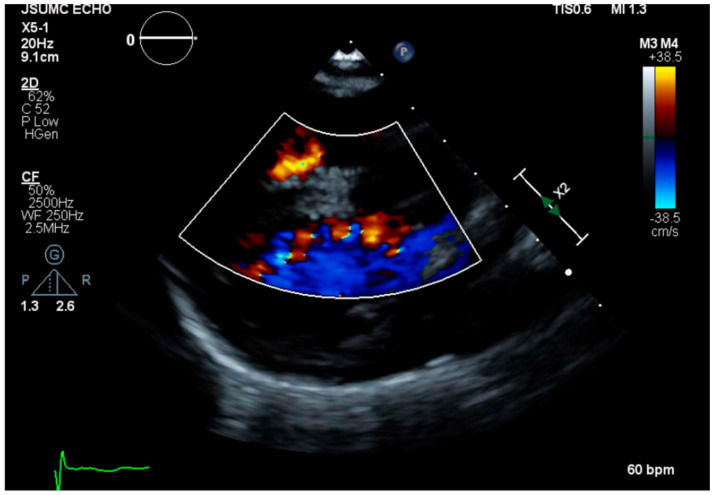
Echocardiogram showing a small muscular ventricular septal defect with left to right shunting.
